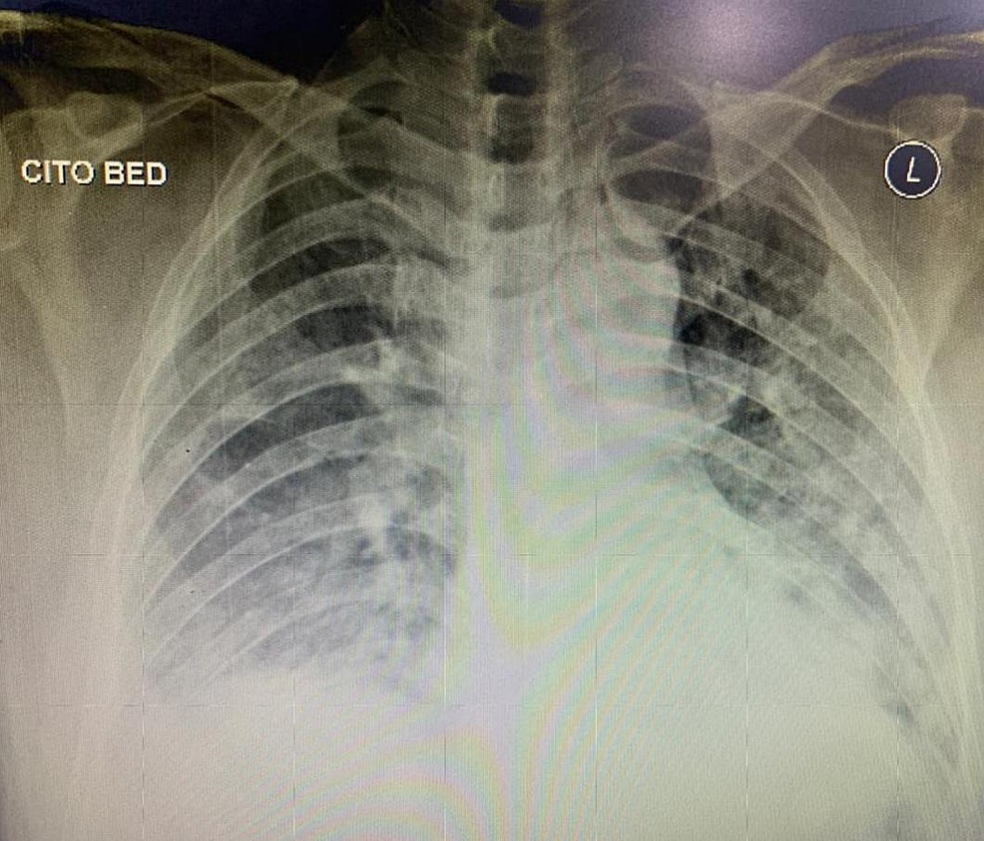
Chest roentgen on admission day showed bilateral infiltrate on both lungs
